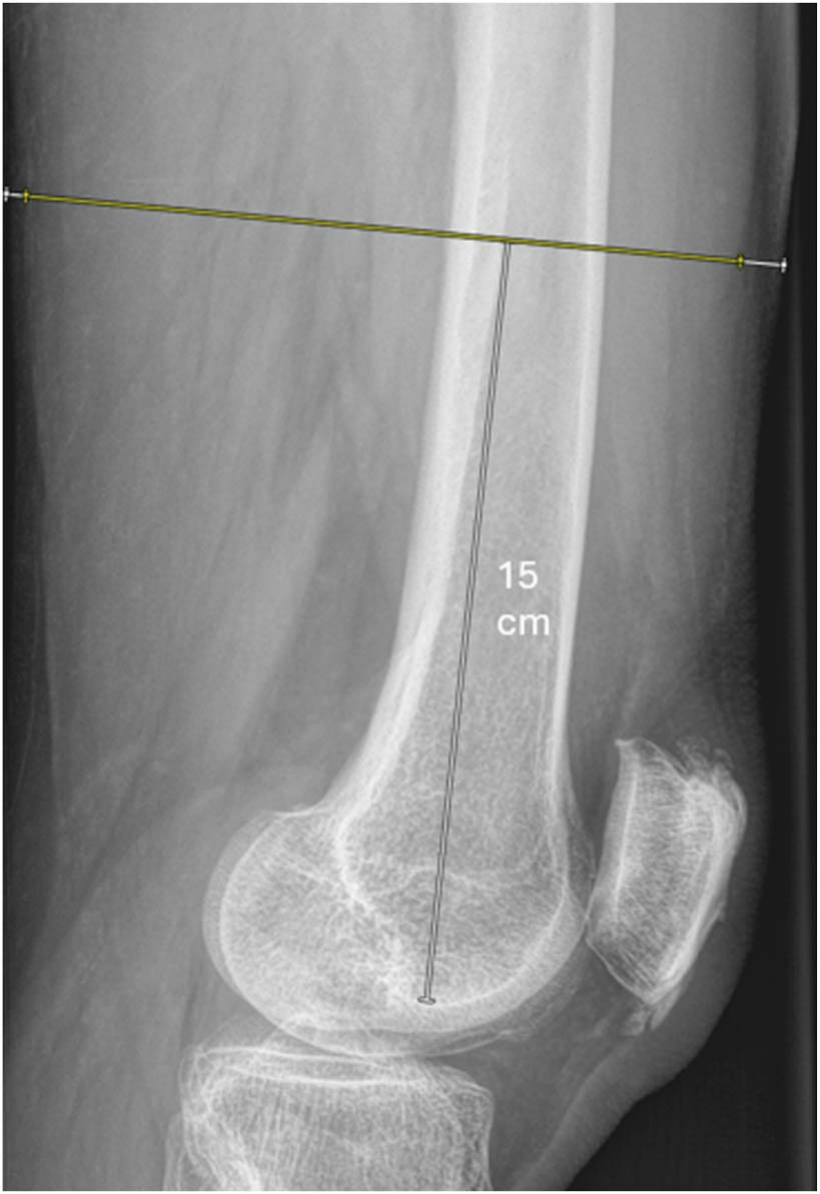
Example of how thigh muscle diameter measurements were obtained from the lateral radiograph. Diameter of thigh muscle (yellow line) and diameter of whole soft tissue envelope (yellow + white lines) were measured 15 cm proximal to the distal terminus of the Blumensaat line.
